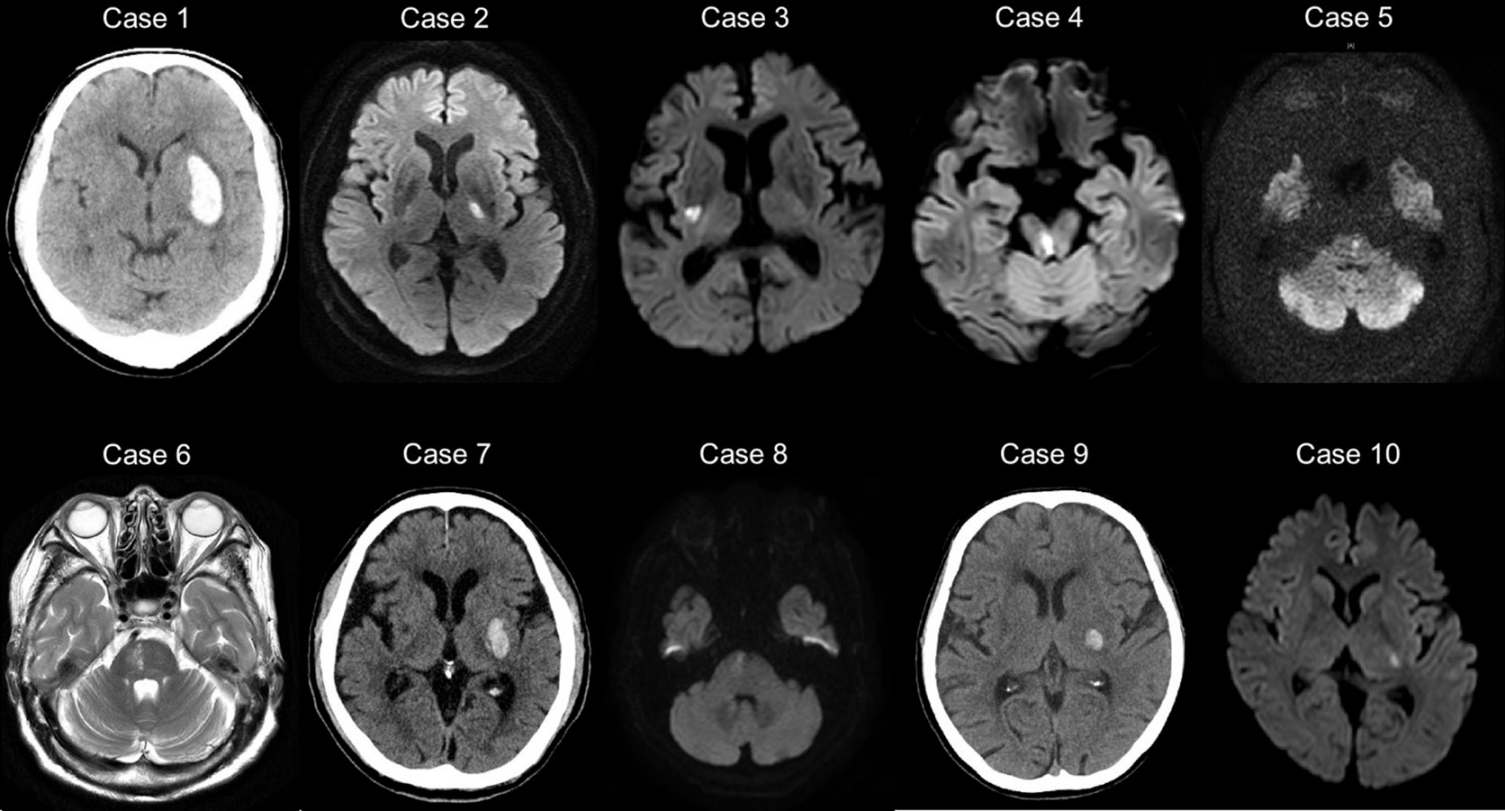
MRI/CT images presenting the lesion sites of 10 post-stroke patients.
